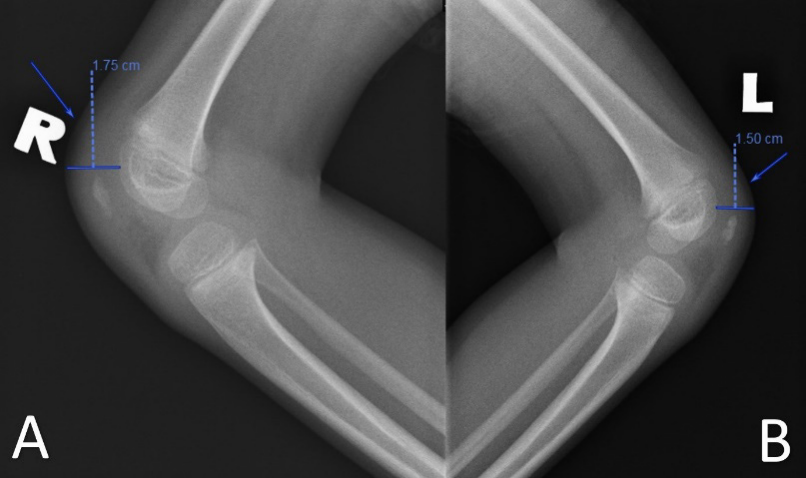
Right (A) and left (B) knee lateral radiographs and the marked area (arrow) of suprapatellar soft tissue swelling and the measurements.
